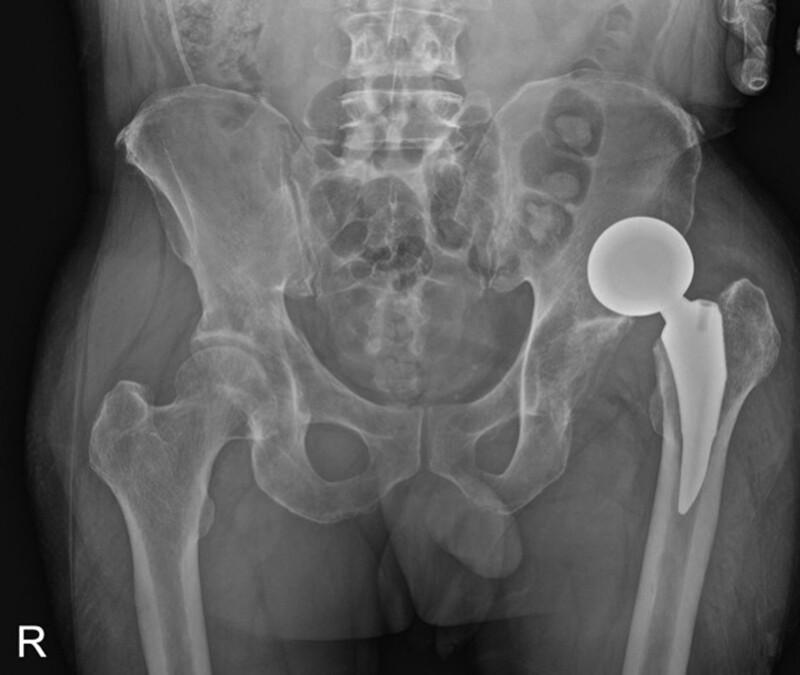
Anteroposterior radiograph demonstrating the first dislocation in hip hemiarthroplasty. The femoral head component appeared as an “O”-shaped concentric circle.
